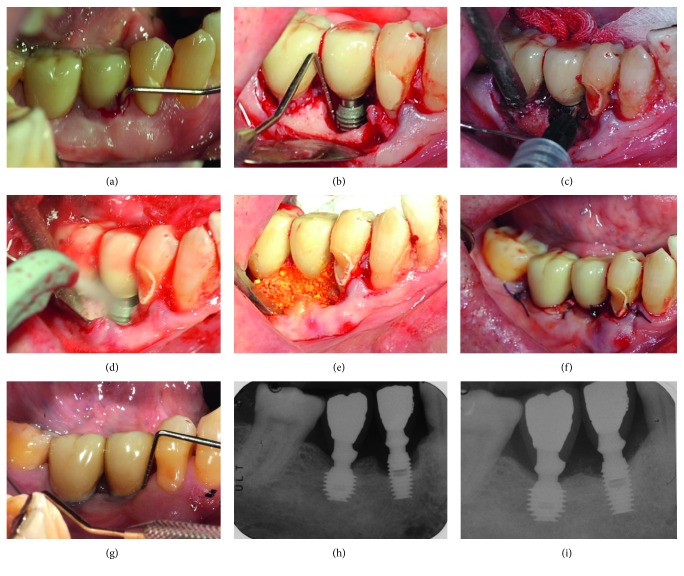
Regenerative treatment of peri-implantitis around an implant placed in the posterior lower jaw of a male patient. (a) Clinical probing before treatment. (b) Intraoperative view of peri-implant bone defect. (c) Application of desiccant. (d) Decontamination with low abrasive air powder. (e) Filling of the peri-implant bone defect. (f) Postoperative clinical aspect. (g) Clinical follow-up 4 years after surgical treatment. (h) Preoperative X-ray. (i) 4-year follow-up.
